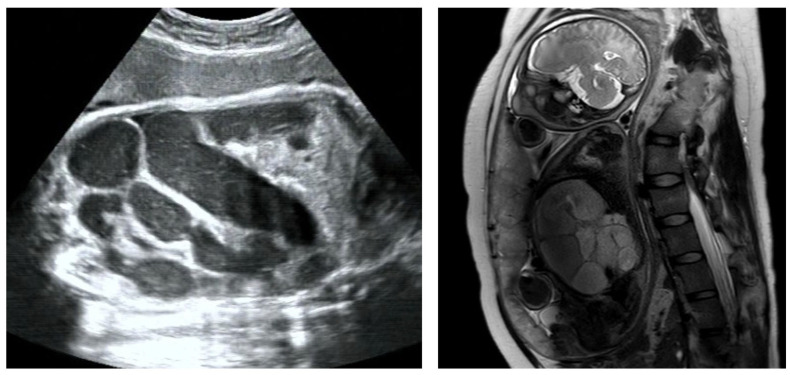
Bowel dilation in ultrasound and MRI examination.
